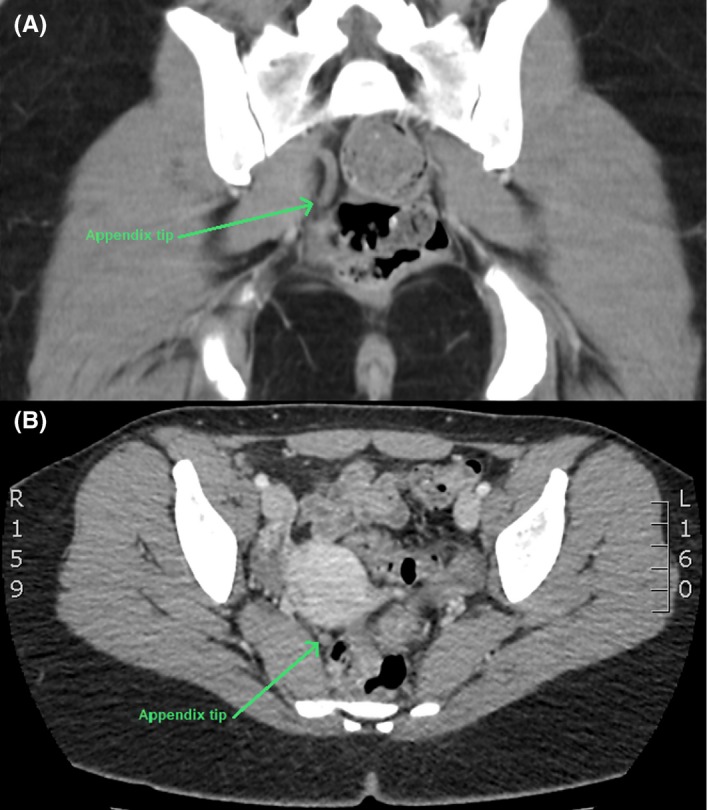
CT Abdomen: (A) Coronal View; (B) Axial View
